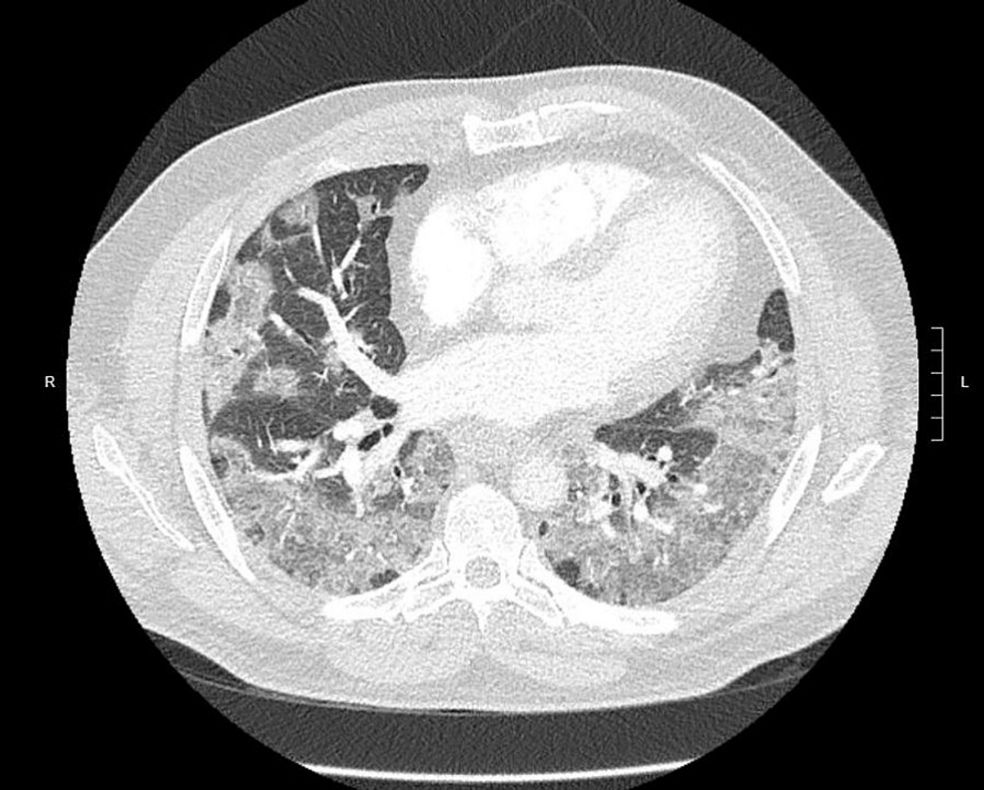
CT chest showing dense bilateral pulmonary infiltrates
